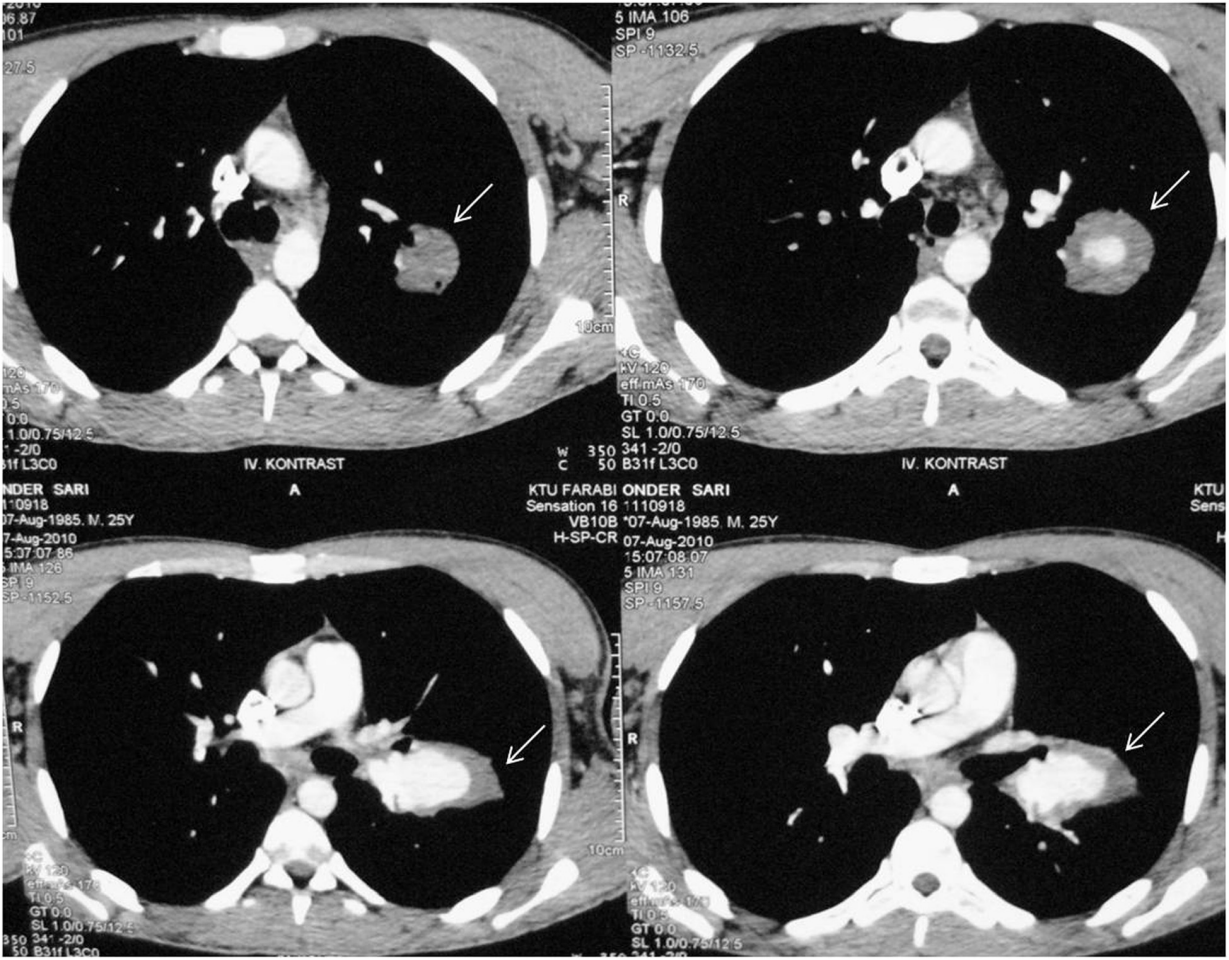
Thorax CT scans are showing the aneurysmatic dilatations and in situ thrombosis in the left pulmonary artery (white arrows).
